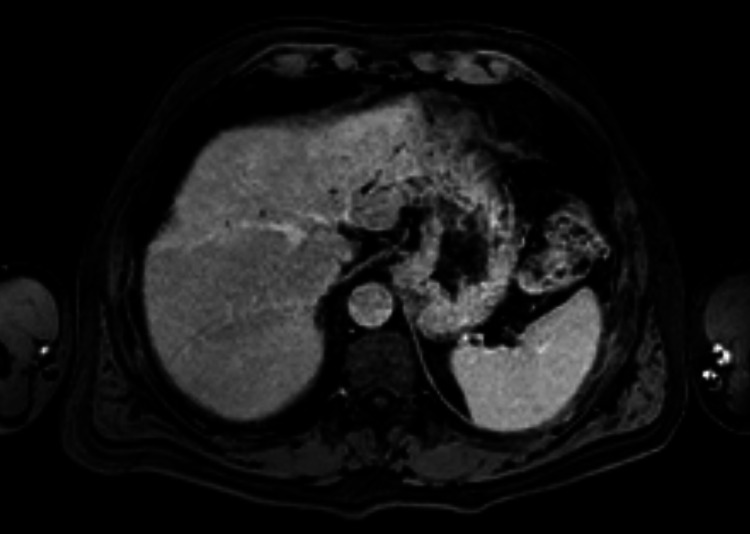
MRI shows in the cranial planes the lesion abuts the left suprahepatic vein (axial T1w post-Gd delayed phase). T1w post-Gd: T1-weighted post-gadolinium
